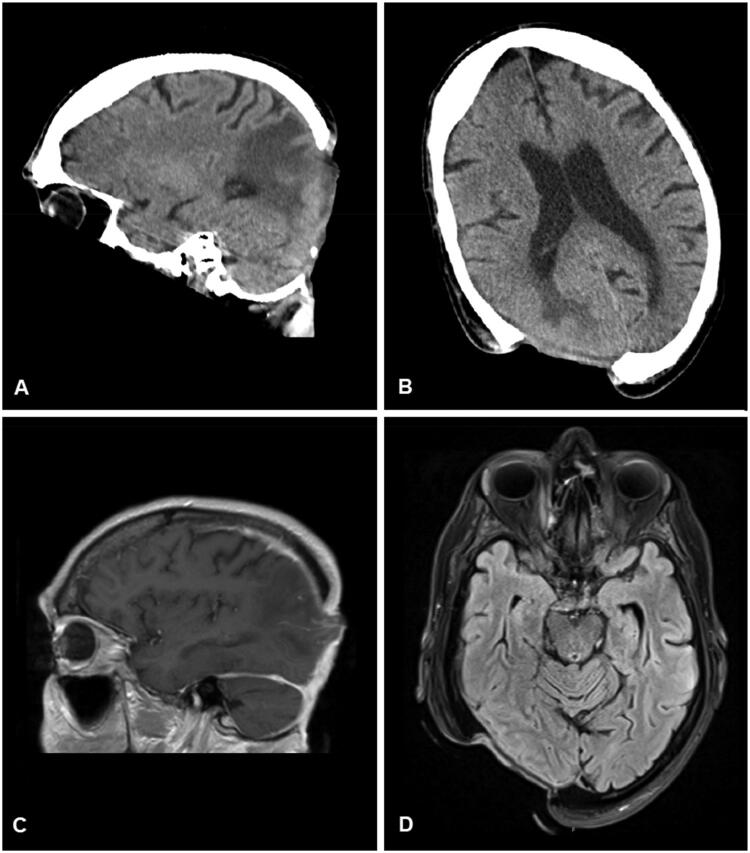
Computed tomographic (A and B) and magnetic resonance imaging (C and D) visualization of the skull defect.
